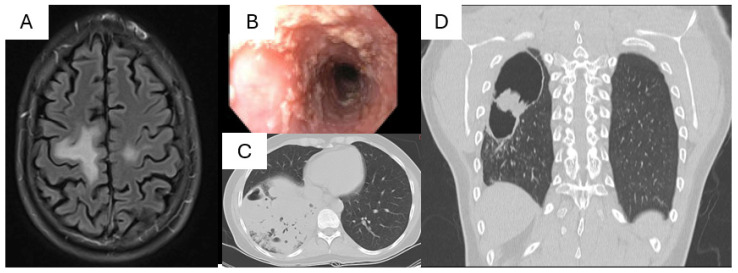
Clinical images of STAT1 GOF and STAT3 HIES: (A) brain MRI showing white matter changes due to John Cunningham virus-related progressive multifocal leukoencephalopathy (PML) in a 30-year-old male with STAT1 GOF; (B) esophageal candidiasis seen in an endoscopy of a 34-year-old male with STAT1 GOF; (C) *S. aureus* lobar pneumonia in a 40-year-old woman with STAT3 HIES; and (D) pneumatocele complicated by an aspergilloma in a 24-year-old male with STAT3 HIES.
